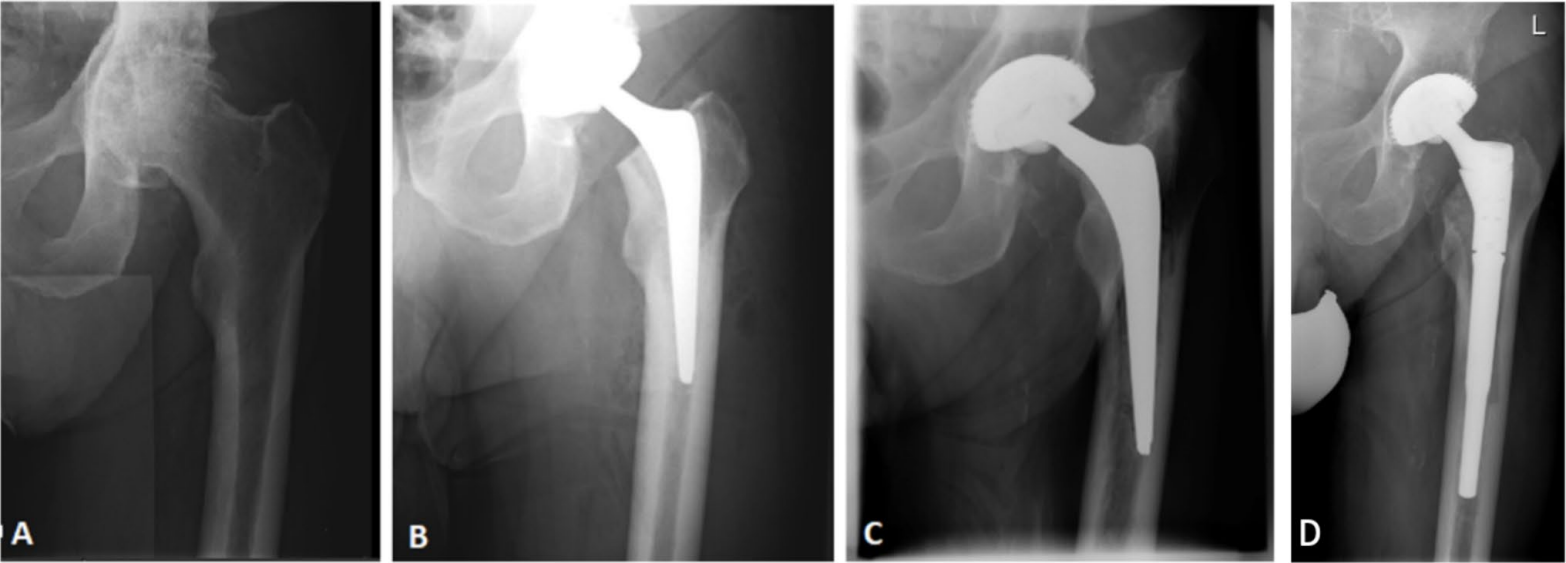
A Pre-operative B 6 weeks post-operative C 9 years post-operative and D post-revision anteroposterior radiographs of the left hip of a 74-year-old male with a renal transplant. The revision surgery was performed nine years after the initial procedure due to aseptic loosening
